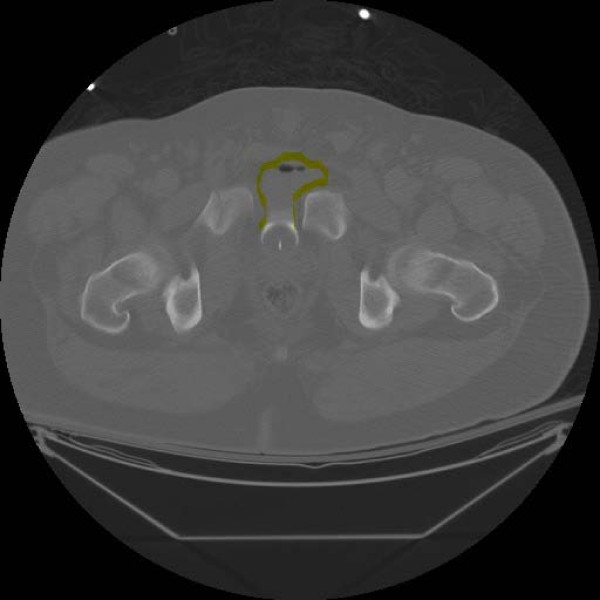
Presenting representative axial pelvic CT cut showing herniation of the urinary bladder into the diastatic symphysis. The herniated bladder has been outlined for clarity.
